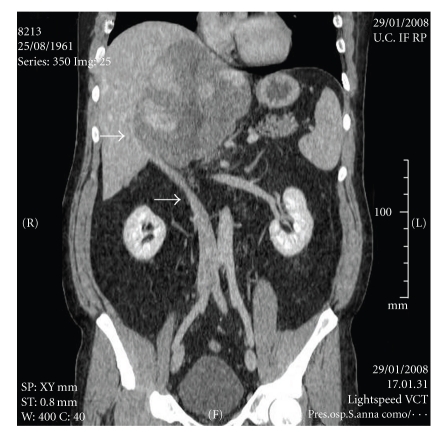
Pre-operative CT scan (coronal view) of the huge hepatic tumour with compression and right dislocation of the interior vena cava (arrows).
